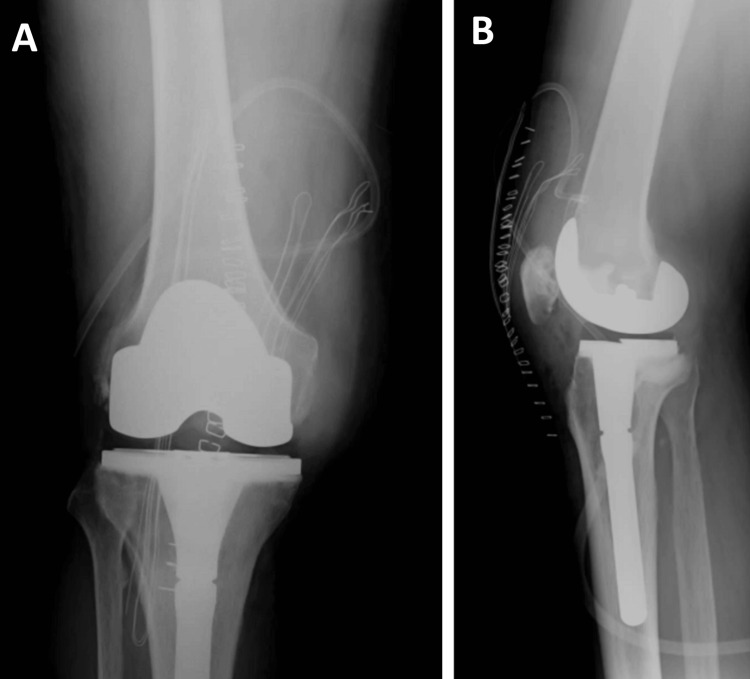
Postoperative radiography of the right knee Coronal view (A), sagittal view (B)
